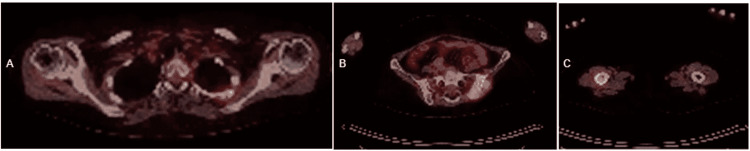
Hypermetabolic lesion on PET scan A: Hypermetabolic lesions located in the left transverse process of T1 are observed. The eft 3rd costal arch, left 4th costal arch, and the vertebral body of T2, are all seen with a tumor appearance;  B: Hypermetabolic lesions located in the left iliacus, left sacroiliac joint (standardized uptake value [SUV]max 13.6), seen with a tumor appearance;  C: Hypermetabolic lesion located in the middle third of the right femur with a tumor appearance PET: Positron emission tomography
